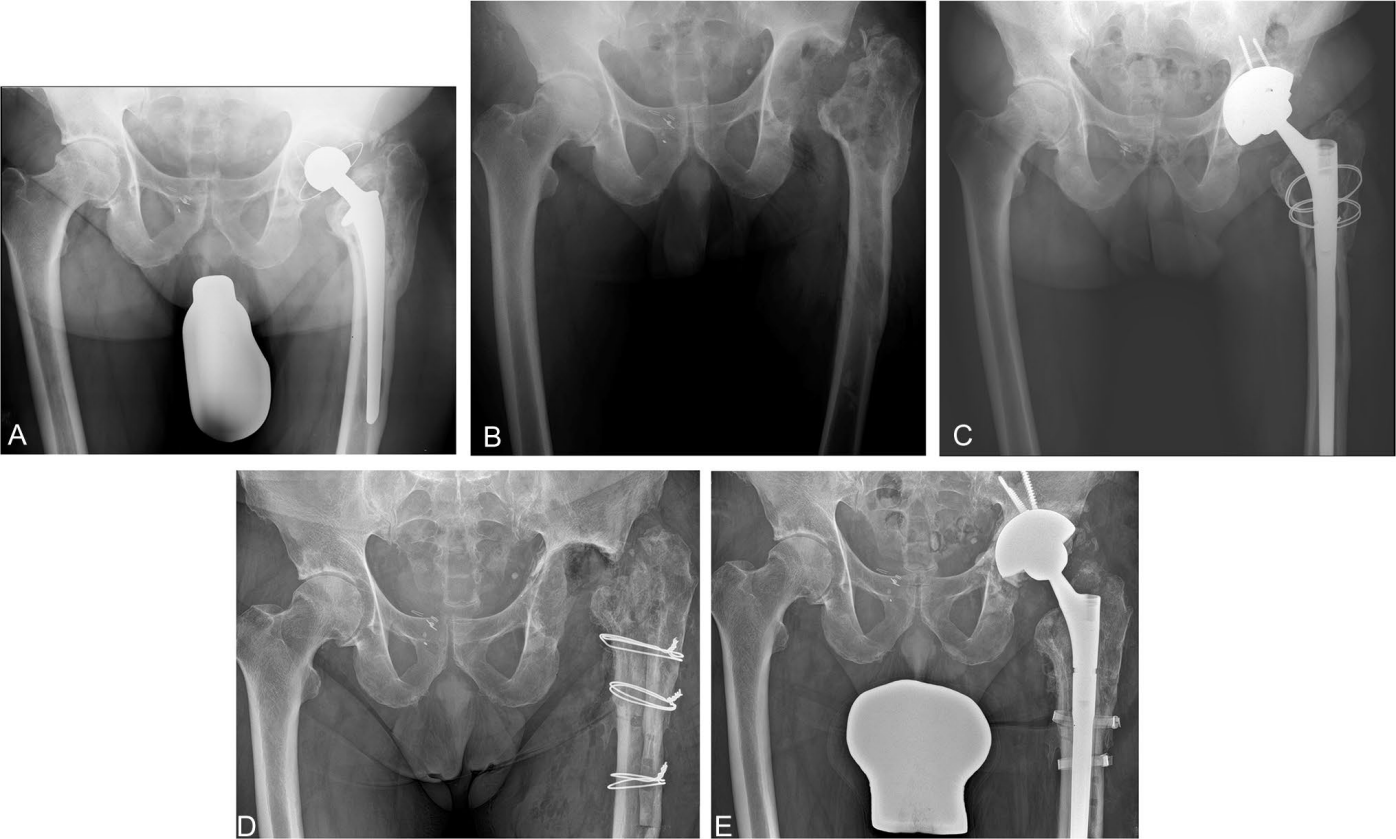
66-year-old male patient with chronic periprosthetic joint infection (PJI) of the left hip. A Anteroposterior view prior to two-stage exchange of the cemented total hip arthroplasty (THA) with loosening of the femoral component. B Radiograph following THA removal showing a Paprosky type 3B femoral defect and type 2B acetabular defect. C Radiograph showing the THA reimplantation after an interim period of 8 weeks using a highly porous metal shell and a modular, tapered fluted stem and two cerclage wires. D Radiograph following resection arthroplasty utilizing an extended trochanteric osteotomy after the patient suffered from reinfection at 35 months. E Radiograph showing the THA reimplantation again with a cementless revision stem; at 80-month follow-up the patient had no signs of PJI and a modified Harris hip score of 85 points
